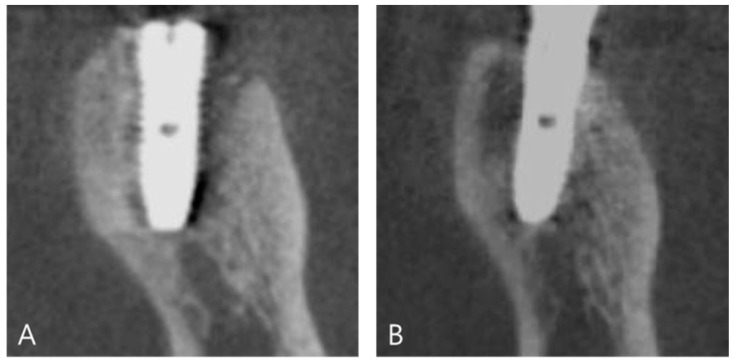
Cone-beam computed tomography after guided bone regeneration using a dentin-derived-barrier membrane (DDB). (A) Coronal plane of cone-beam computed tomography immediately after implant placement with guided bone regeneration using a DDB. There was a radiolucent defect that was filled with auto-DDM and covered with a DDB. (B) At 4 years and 8 months, the cortical bone around the implant neck was fully repaired and supported by well-developed corticocancellous bone.
